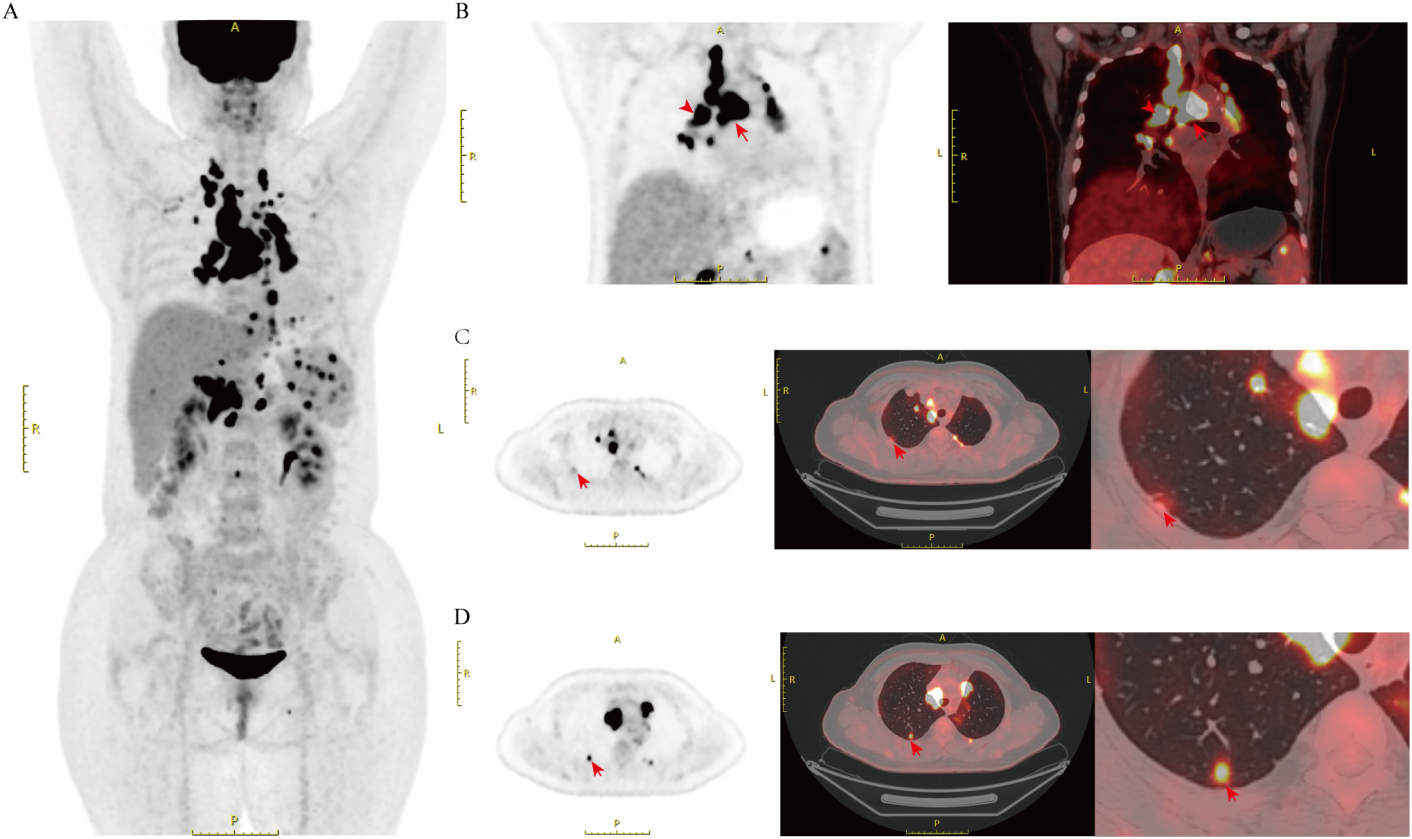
PET/CT imaging of the patient before steroid treatment (A) PET/CT imaging of Systemic Sarcoidosis. (B) PET/CT imaging of hypermetabolic lymph nodes in the mediastinum and hilum, some forming a confluent mass, with SUV max values of 17.2 and 21.5(arrows). (C) A markedly hyperintense lesion can be observed along the pleural surface (arrows). (D) Arrows point to the hypermetabolic lesions in the right upper lobe, with the SUV max of 6.8. SUV max, Standardized Uptake Value maximum.
